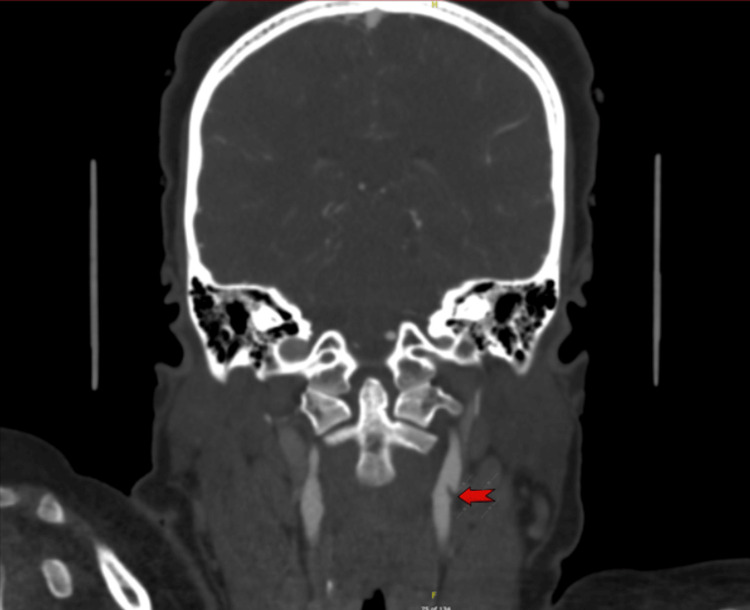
CT angiography (Coronal view) Red arrow showing irregular hypodensity at the left internal carotid artery origin, suggestive of a web or plaque.
